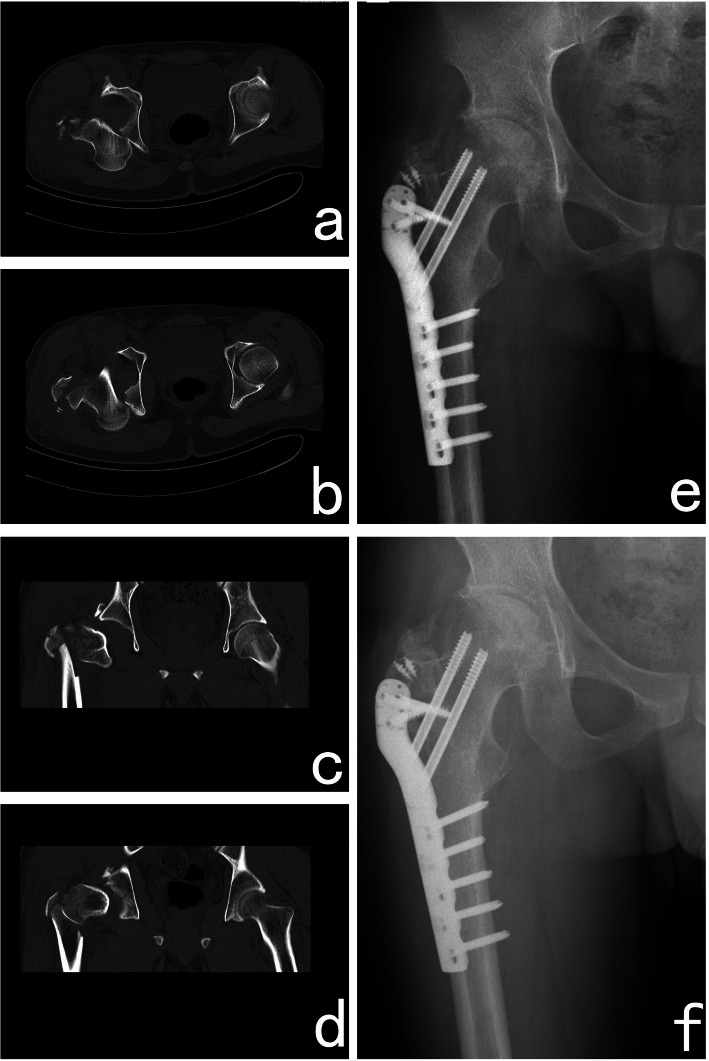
Case 17. A 28-year-old male patient had a traumatic acetabular fracture combined with an ipsilateral intertrochanteric fracture in his right hip. The patient underwent open reduction and internal fixation surgery for both his acetabulum and proximal femur. An absorbable screw was used to fix the fracture fragment of the posterior wall. Plates and screws were used to fix the intertrochanteric fracture. a–d Computed tomography image showing a posterior wall fracture of the acetabulum and dislocation of the hip joint. e Anterior-posterior view at the final follow-up. f Lateral view before hip arthroplasty at the final follow-up
